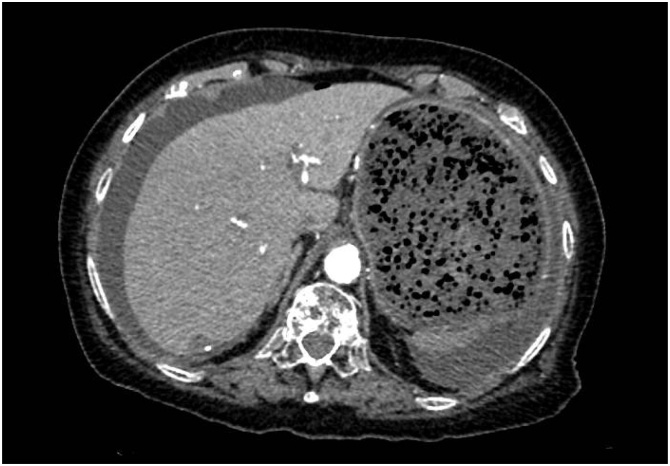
CT scan showing stomach dilatation.
